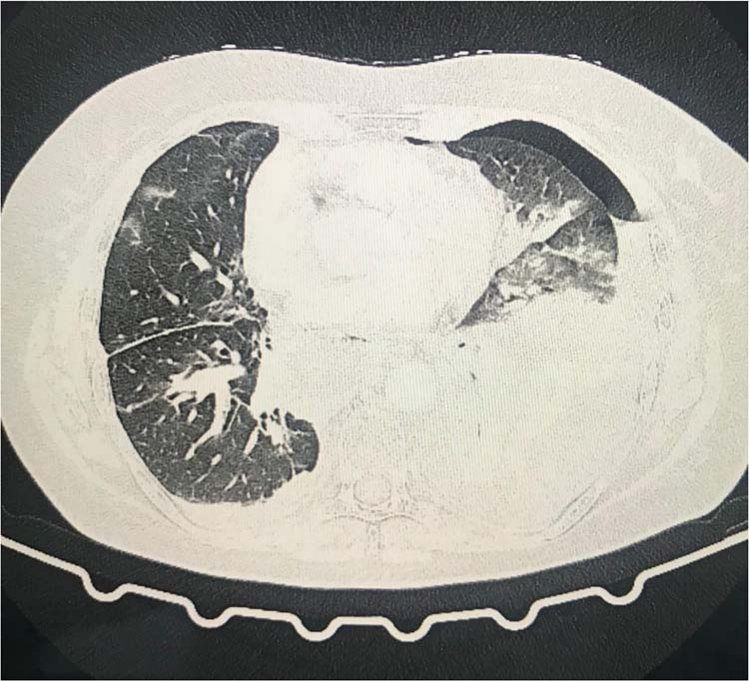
Second day follow-up showed a hydropneumothorax on the left chest CT scan.
